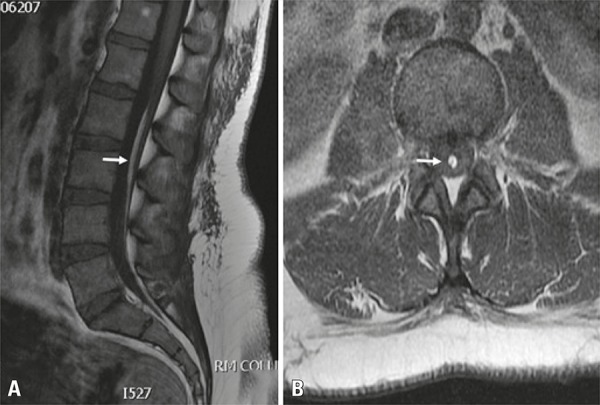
Pre-operative images. T1-weighted magnetic resonance images showing a lipoma of the *filum terminale* (hyperintense sign within the *filum terminale*, white arrow) in sagittal (A) and axial (B) planes
